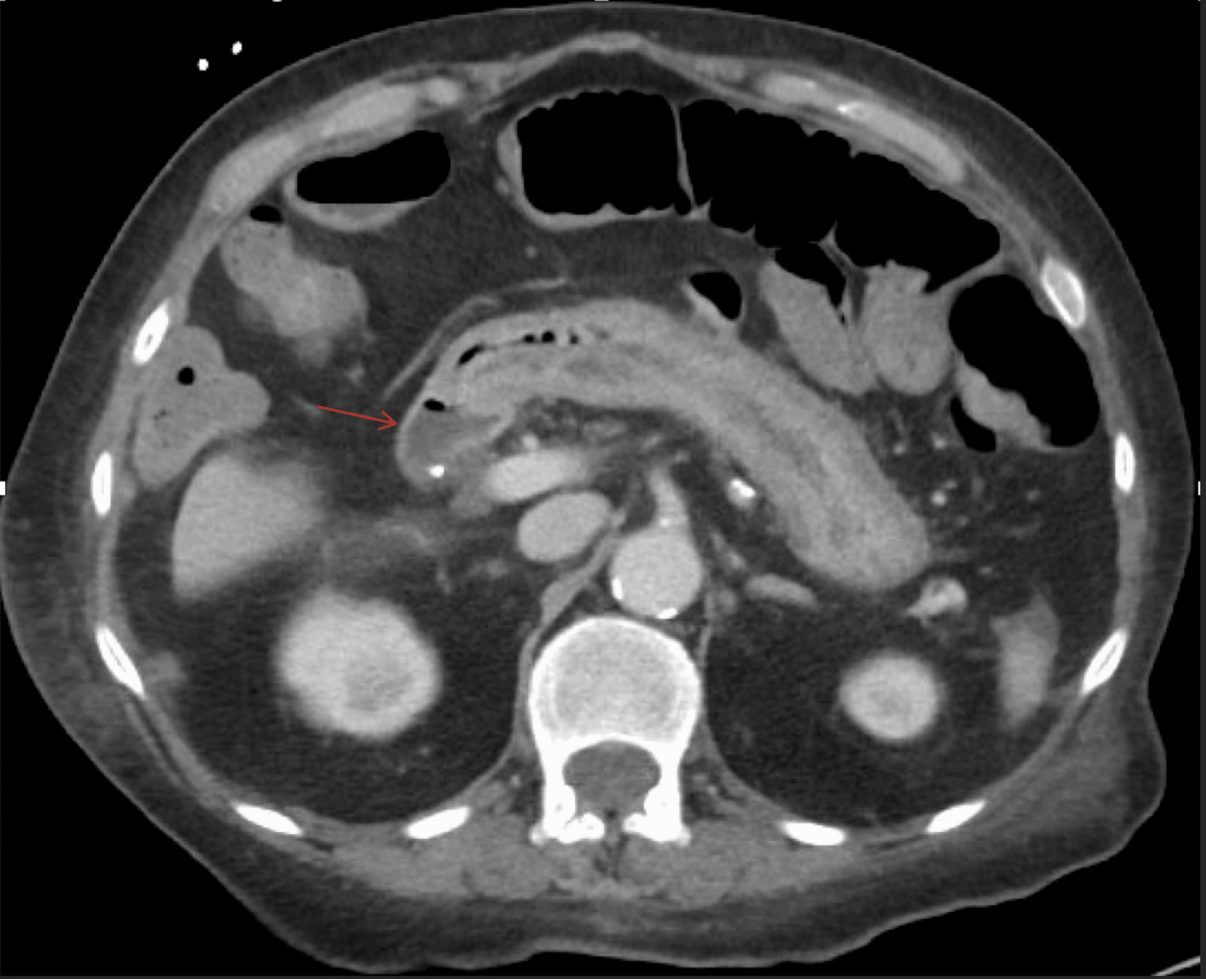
CT abdomen and pelvis with IV contrast (axial view) demonstrates a history of prior gastric bypass surgery with mild distention of small bowel loops on the right side (red arrow). CT: computed tomography; IV: intravenous
